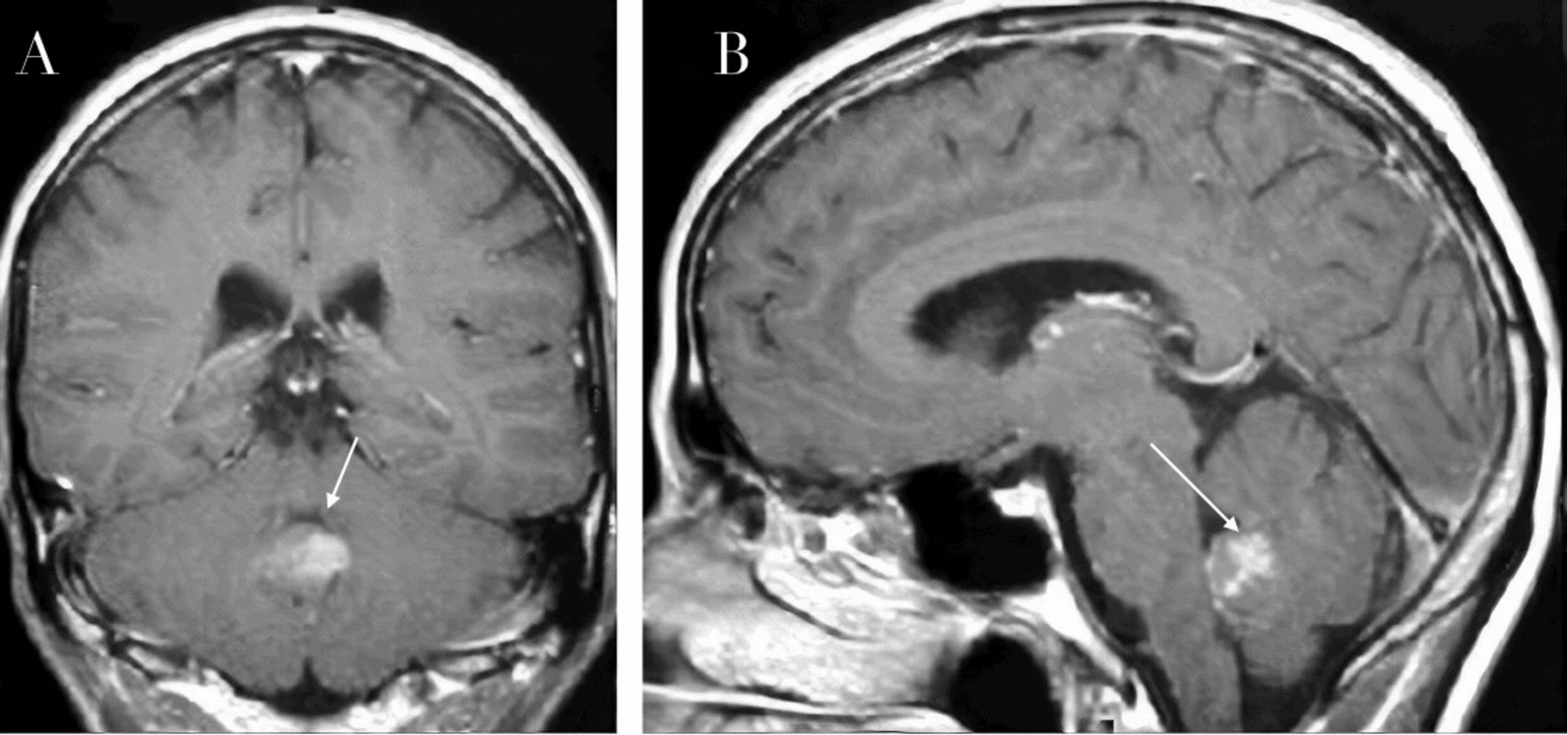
Initial contrast-enhanced brain MRI obtained at hospital admission. A: Coronal T1-weighted post-contrast image demonstrating a heterogeneously enhancing midline posterior fossa mass (white arrow) centered in the cerebellar vermis, measuring approximately 4.2 × 3.8 × 3.5 cm. The lesion shows avid, irregular contrast enhancement. There is marked compression and anterior displacement of the fourth ventricle, resulting in obstructive triventricular hydrocephalus. B: Sagittal T1-weighted post-contrast image confirming a heterogeneously enhancing mass centered in the cerebellar vermis (white arrow). The lesion causes a significant mass effect on the fourth ventricle, with anterior displacement and obstructive hydrocephalus. No evidence of spinal leptomeningeal dissemination was identified on initial staging MRI of the neuroaxis.
